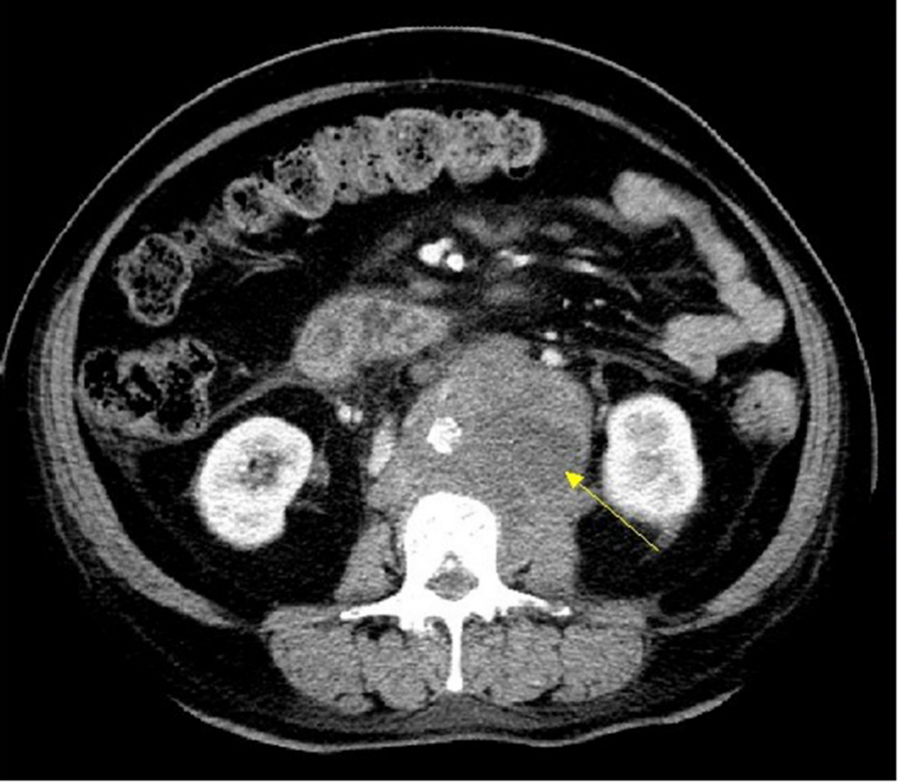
CT scan of abdomen showing a mass. Axial contrast-enhanced CT image shows a large lobulated irregular mass with inhomogenous enhancement that is encasing and anteriorly displacing the abdominal aorta.
